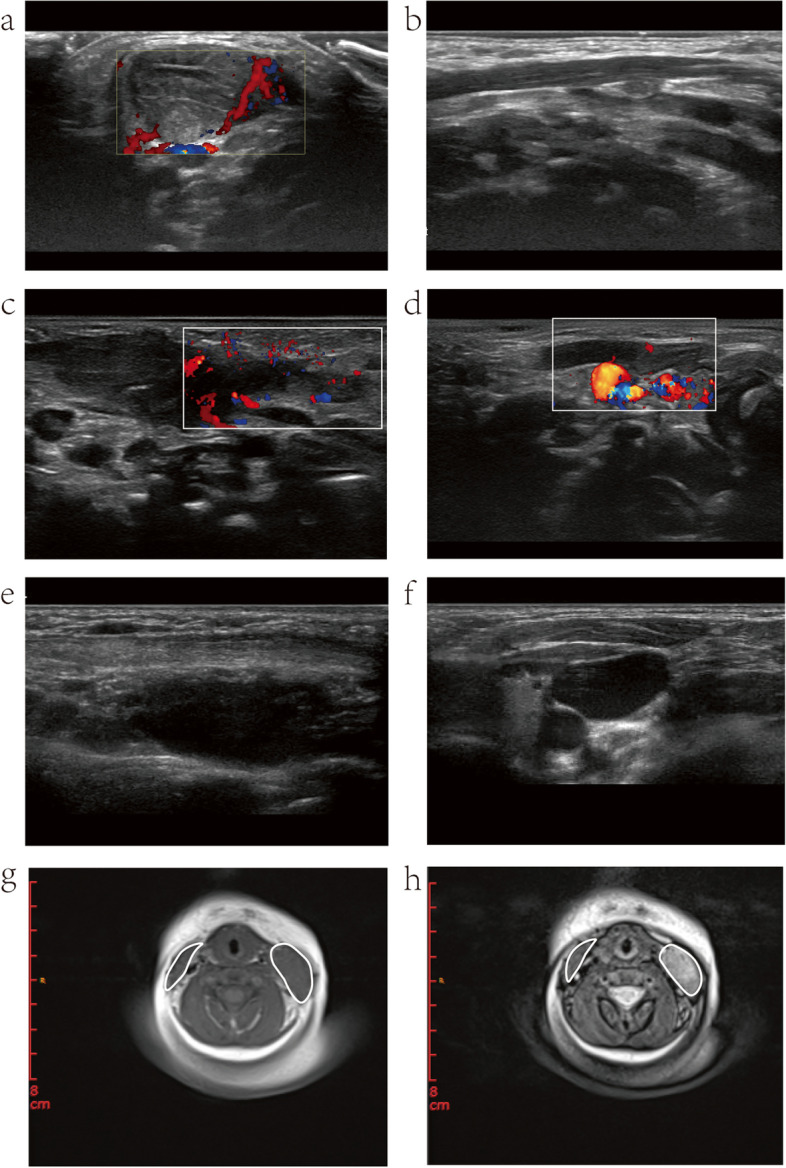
Ultrasound and MRI images of the infant in Case one at initial visit, immediately after MM, and at final follow-up (a) and (b) Ultrasound images of the involved and unaffected sides at initial visit (c) and (d) Instant ultrasound images of the involved and normal sides after MM (e) and (f) Ultrasound images of the involved and normal sides at final follow-up (g) Instant MRI-T1_tse images after MM (h) Instant MRI-T2_tse images after MM
